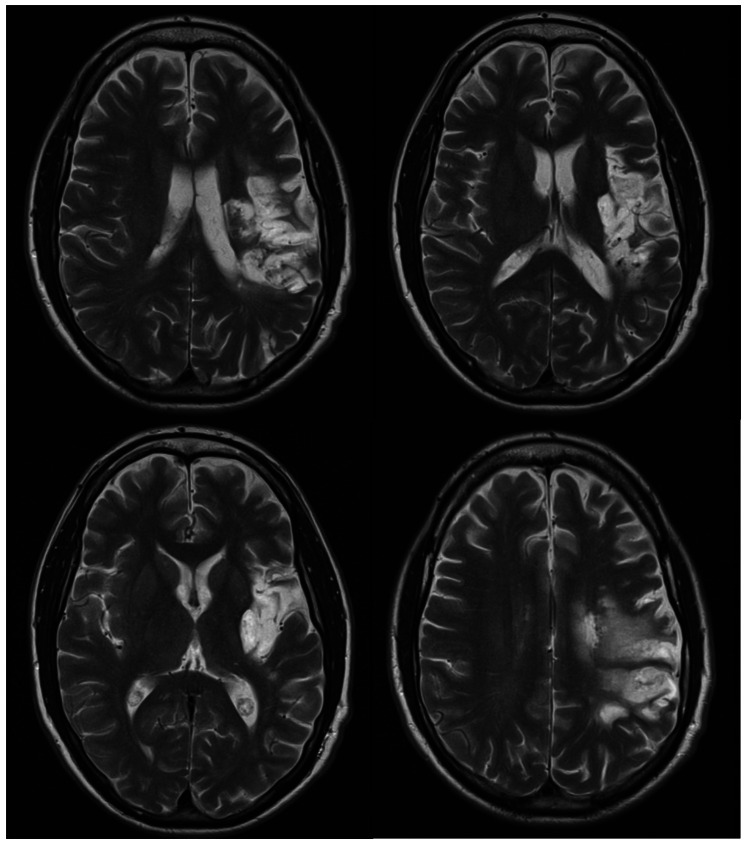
Interval Axial T2 MRI scan performed 5 months following acute stroke. Indicating mature large left MCA territory infarct
